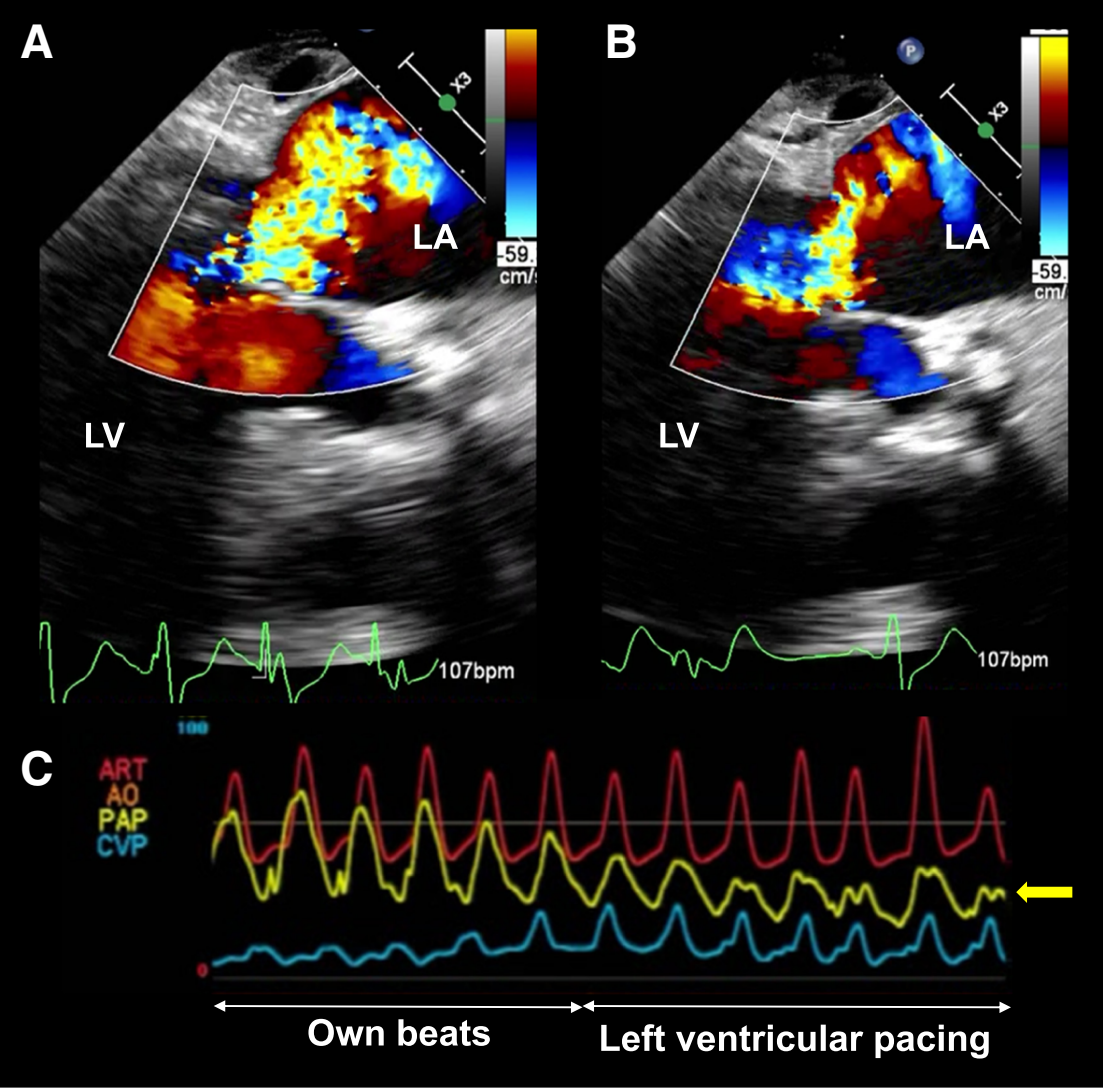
Intraoperative findings. Transesophageal echocardiography showing severe mitral regurgitation (a), which improved by left ventricular pacing (b). Monitoring results showing the decrease in pulmonary arterial pressure with left ventricular pacing (c). The yellow line (arrow) indicates the pulmonary arterial pressure. LA left atrium, LV left ventricle
